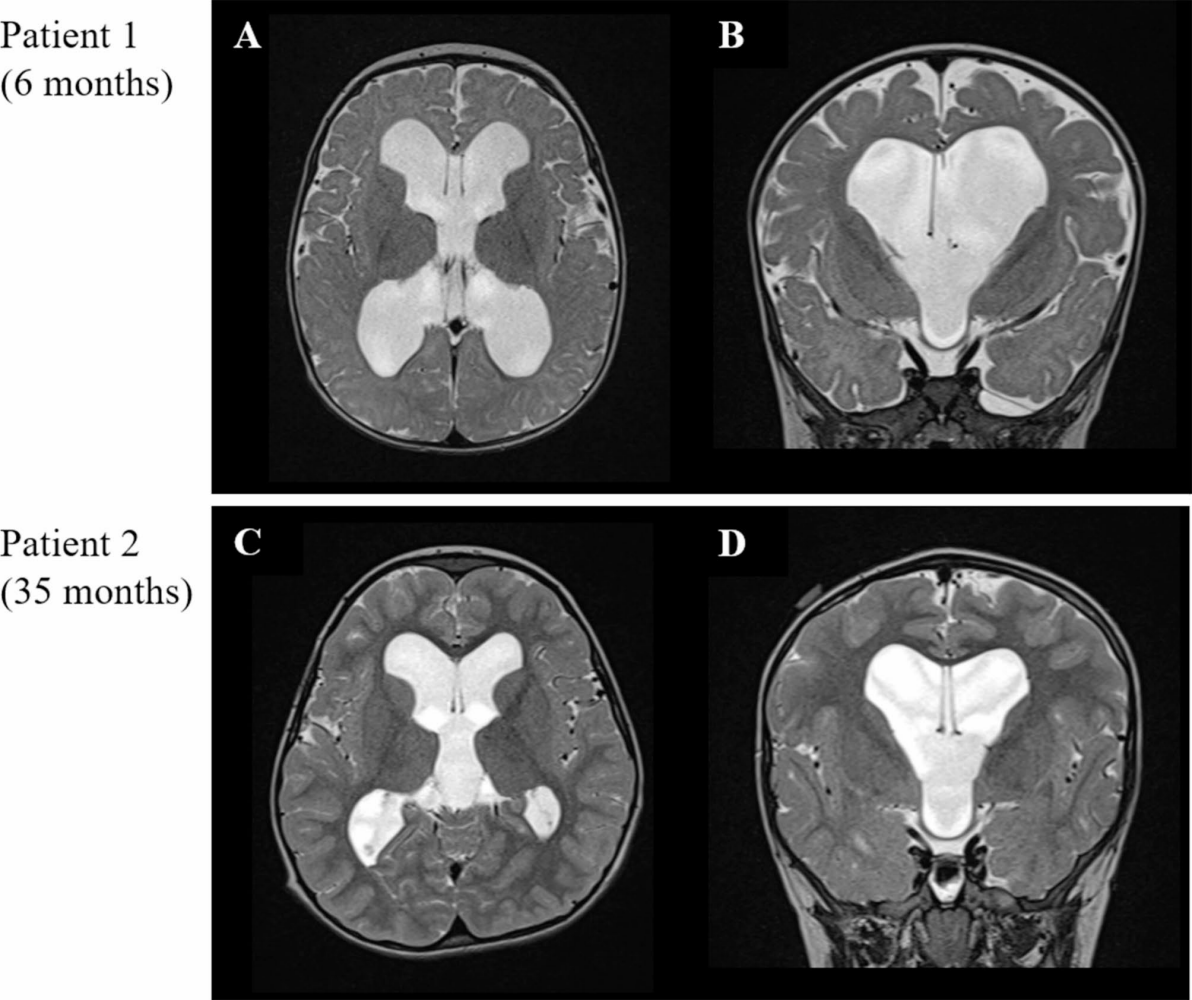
Brain magnetic resonance imaging (MRI) was taken just before the operation in two achondroplasia patients who underwent ventriculoperitoneal shunt. Each was performed at 6 months (patient 1, A, B) and 35 months (patient 2, C, D) of age. Both patients showed marked dilatation of lateral and third ventricles on T2-axial (A, C) and T2-coronal (B, D) images. (A, B) Frontal horn width = 67.85 mm, Evan’s ratio = 0.51 (C, D) Frontal horn width = 61.31 mm, Evan’s ratio = 0.42
